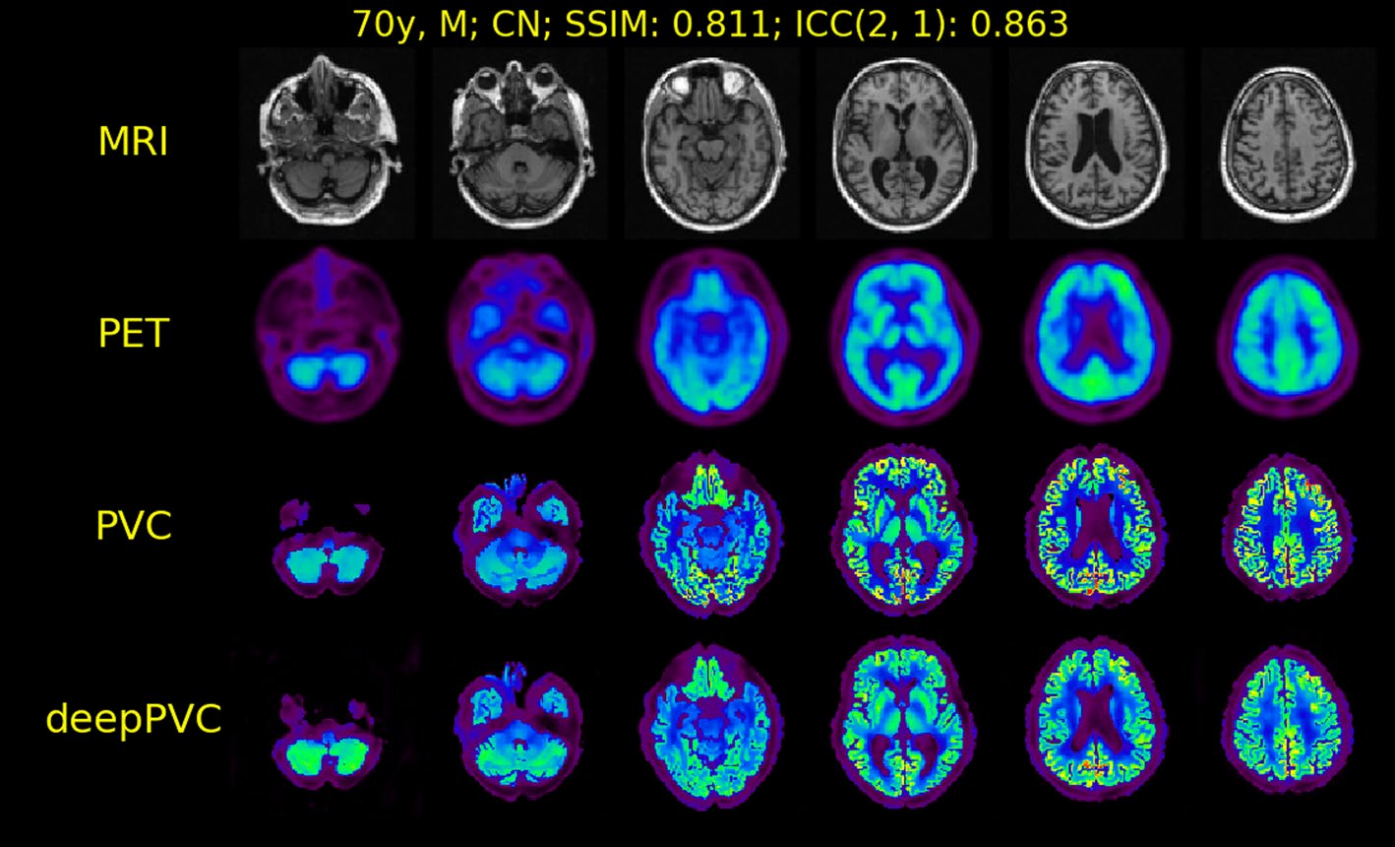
MR, PET, and real and predicted PV-corrected maps for cases with the best ICC among the [^18^F]FDG test data. CN, cognitively normal; MR, magnetic resonance; PET, positron emission tomography; PV, partial volume
